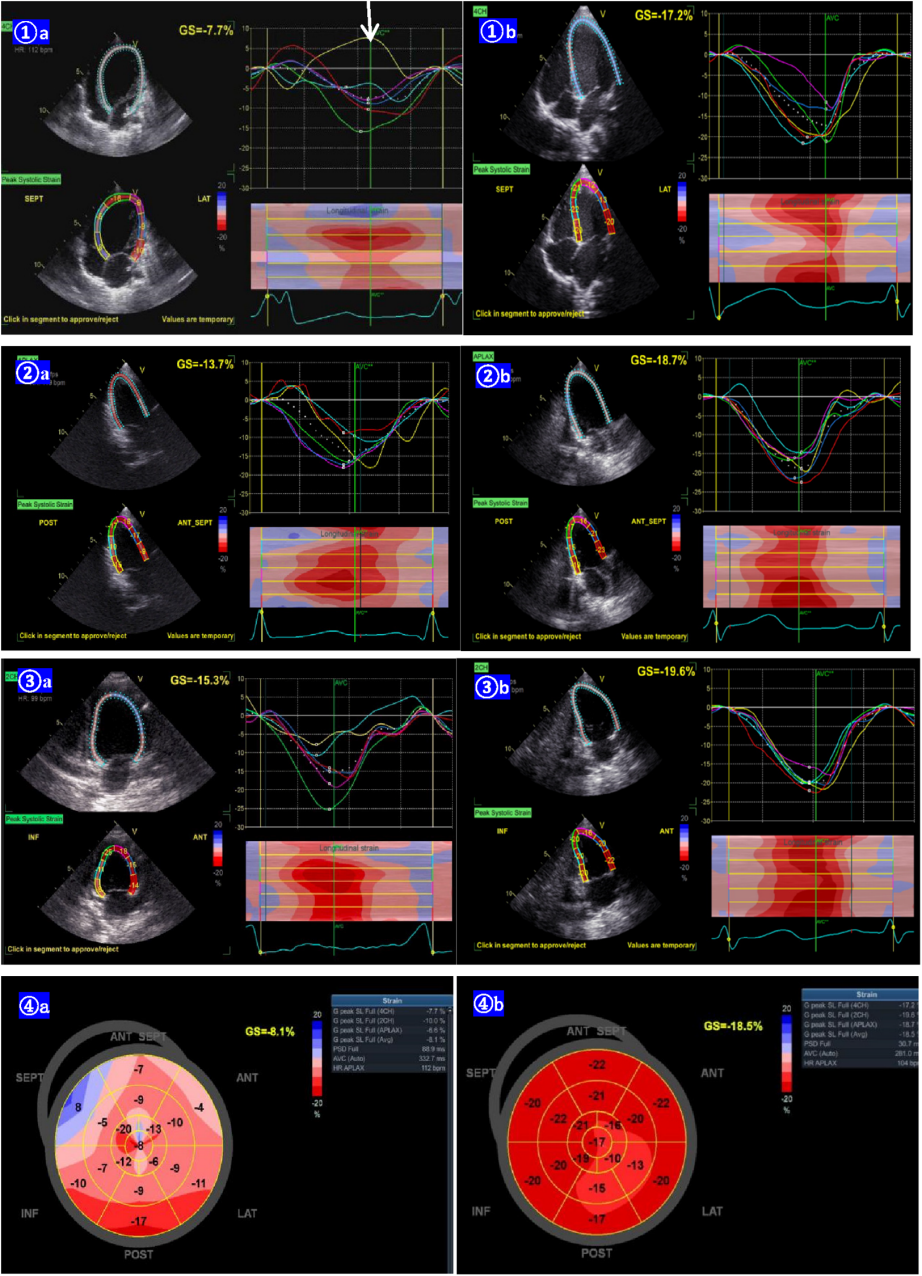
Lv longitudinal strain curves from the apical 4-chamber (1A), 2-chamber (2A), and 3-chamber (3A) view of case 2 before the pharmacotherapy of amiodarone, showing the basal segments of the interventricular septal paradoxical motion (arrow, positive strain). The LV longitudinal strain becomes normal 6 months post pharmacotherapy (1B,2B,3B). The bull's eye of the LV longitudinal strain before the pharmacotherapy, shows the segment of the post-interventricular septal wall paradoxical motion (positive strain). The bull's eye of the LV longitudinal strain changed to normal 6 months after the pharmacotherapy (4B).
